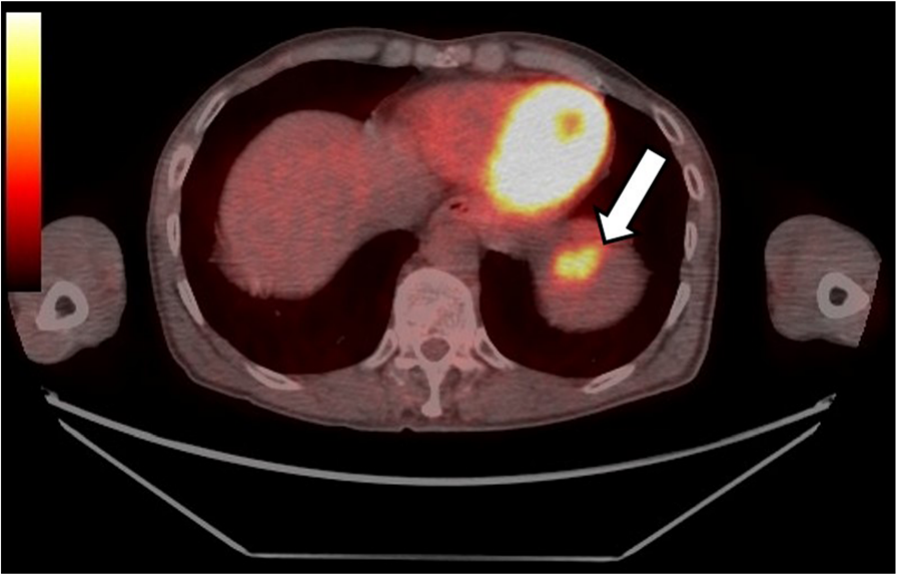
The PET-CT image shows intense fluorine-18 fluorodeoxyglucose (18F–FDG) uptake with a maximum standardized uptake value (SUV) of 9.0 (*arrow*). There is no suspected extrasplenic tumor dissemination or metastasis
